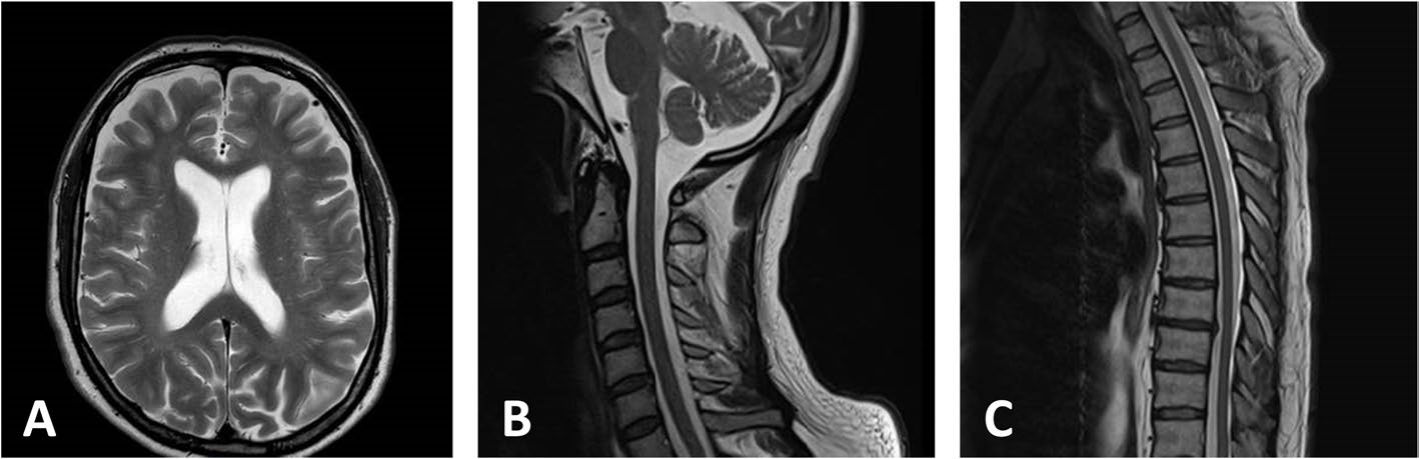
MR imaging findings. MR imaging of the brain and spinal cord did not reveal findings suggestive of myelopathy or multiple sclerosis. A. brain, B. cervical spinal cord, C. thoracic spinal cord. All shown MR images are T2 weighted
